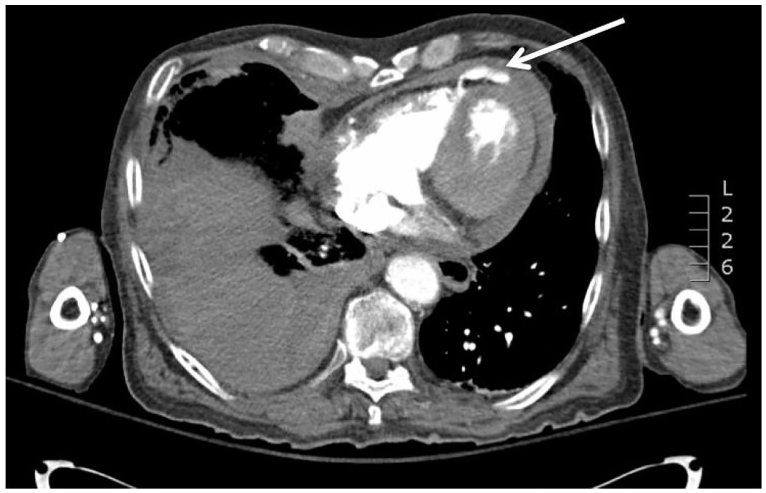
Computed tomography of chest revealing rupture of right ventricle with leak of contrast and associated hemopericardium (white arrow).
